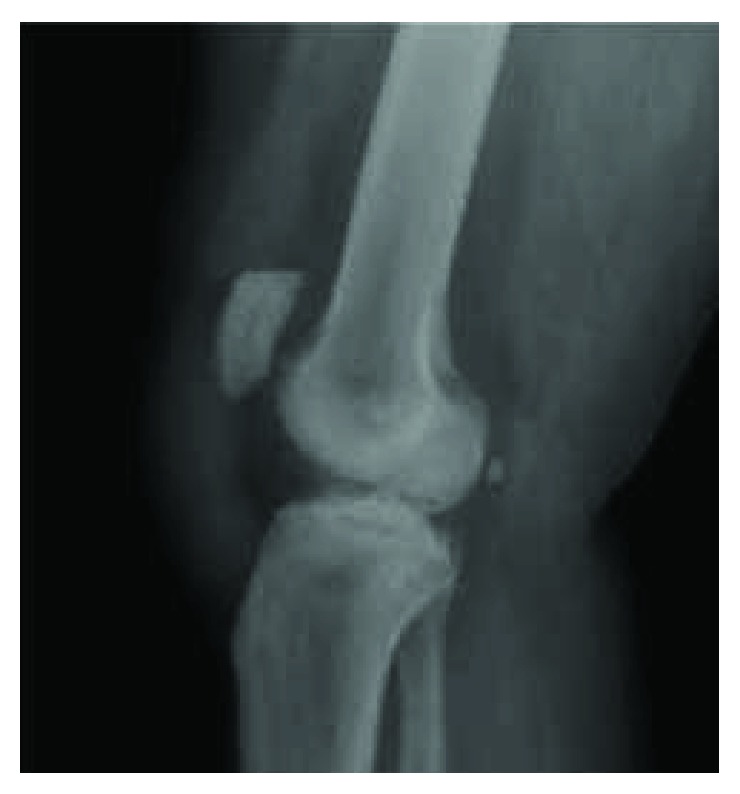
Postoperative lateral X-ray of the patient's right knee demonstrating a corrected patella alta deformity immediately following the first procedure. Insall-Salvati Index = 1.23, Caton-Deschamps Index (CDI) = 1.14.
